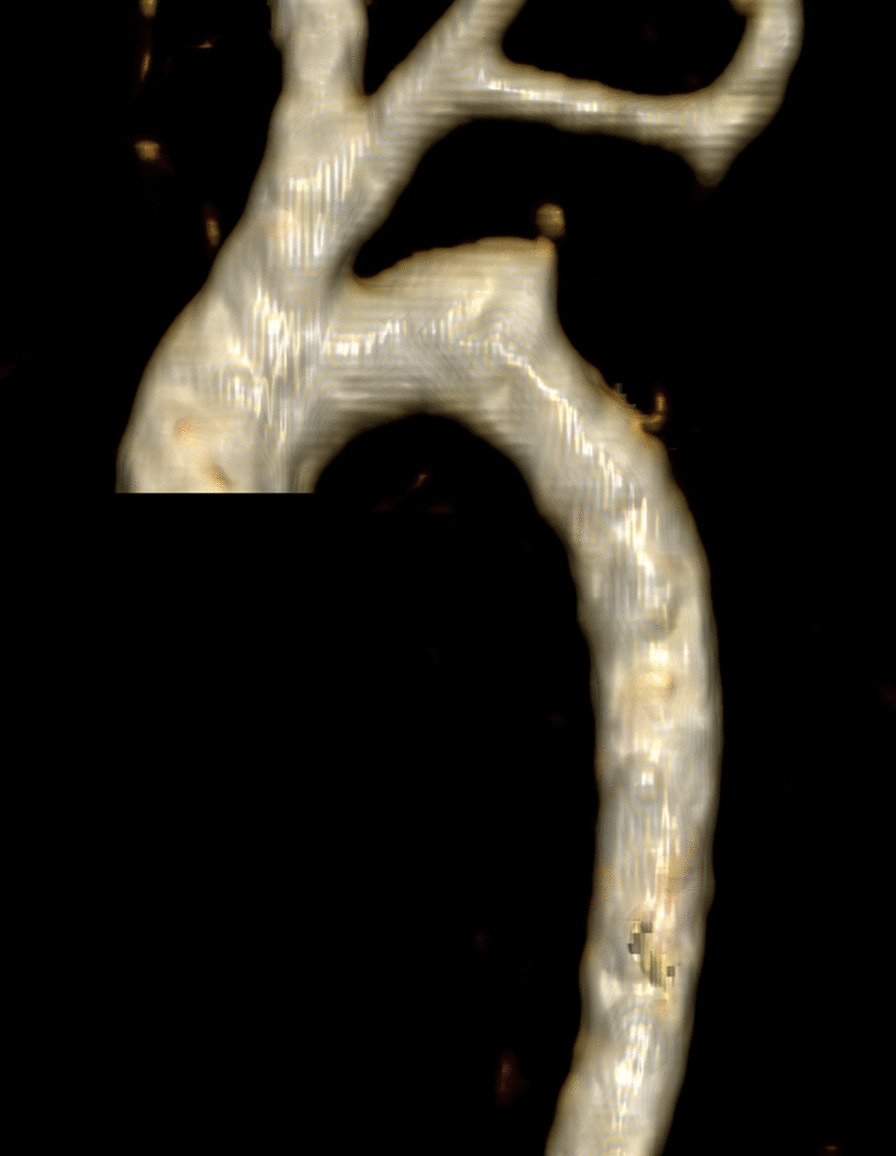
Postoperative three-dimensional computed tomography angiography showing a wide aortic arch without stenosis
